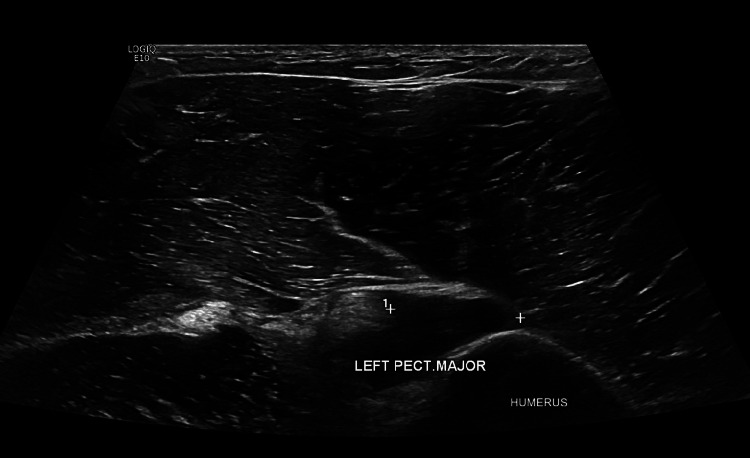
Ultrasound scan of the left shoulder Partial dislodgment of the distal fibers at the musculotendinous aspect of the pectoralis muscle at the humeral insertion with a partial gap of around 15 mm from the humeral attachment was observed.
